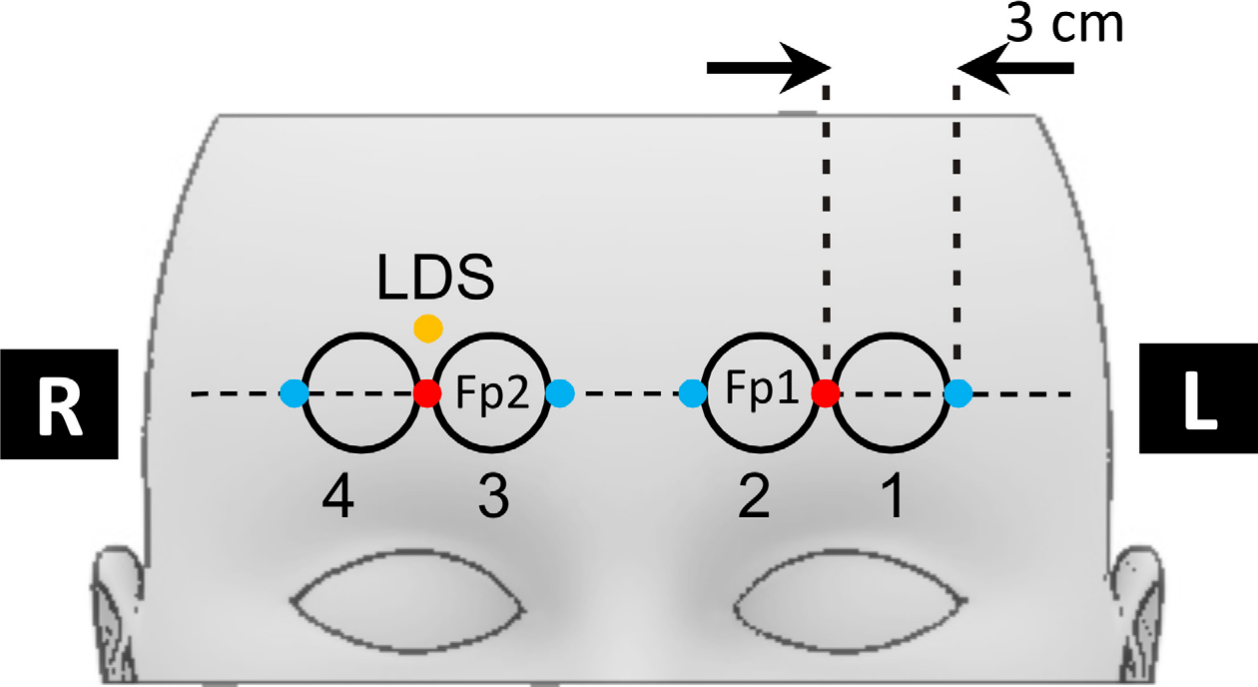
Scheme of the fNIRS optode placement on the forehead. Red dots indicate the positions of fNIRS source optodes, blue dots the positions of the four detector optodes. Numbered circles indicate the locations of the four fNIRS channels. The orange dot indicates the positions of the laser Doppler sensor.
